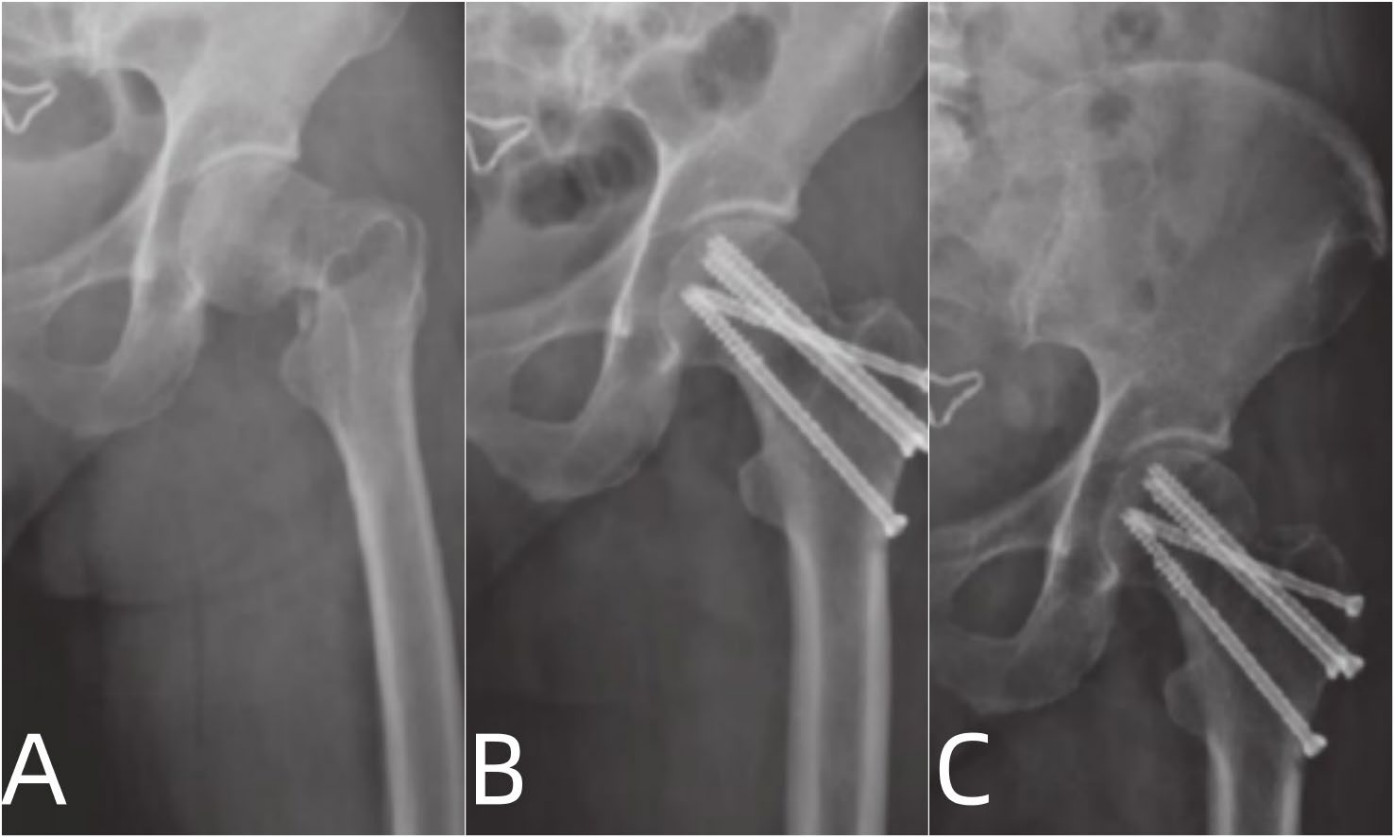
Anteroposterior radiographs of a 37-year-old male patient with a left femoral neck fracture (Pauwels type II, Garden subtype). A shows the radiograph before the operation, B shows the radiograph 1 week after surgery indicating that the fracture was in a good position for reduction and internal fixation, and C exhibits radiograph 6 months after surgery showing a reasonable prognosis
